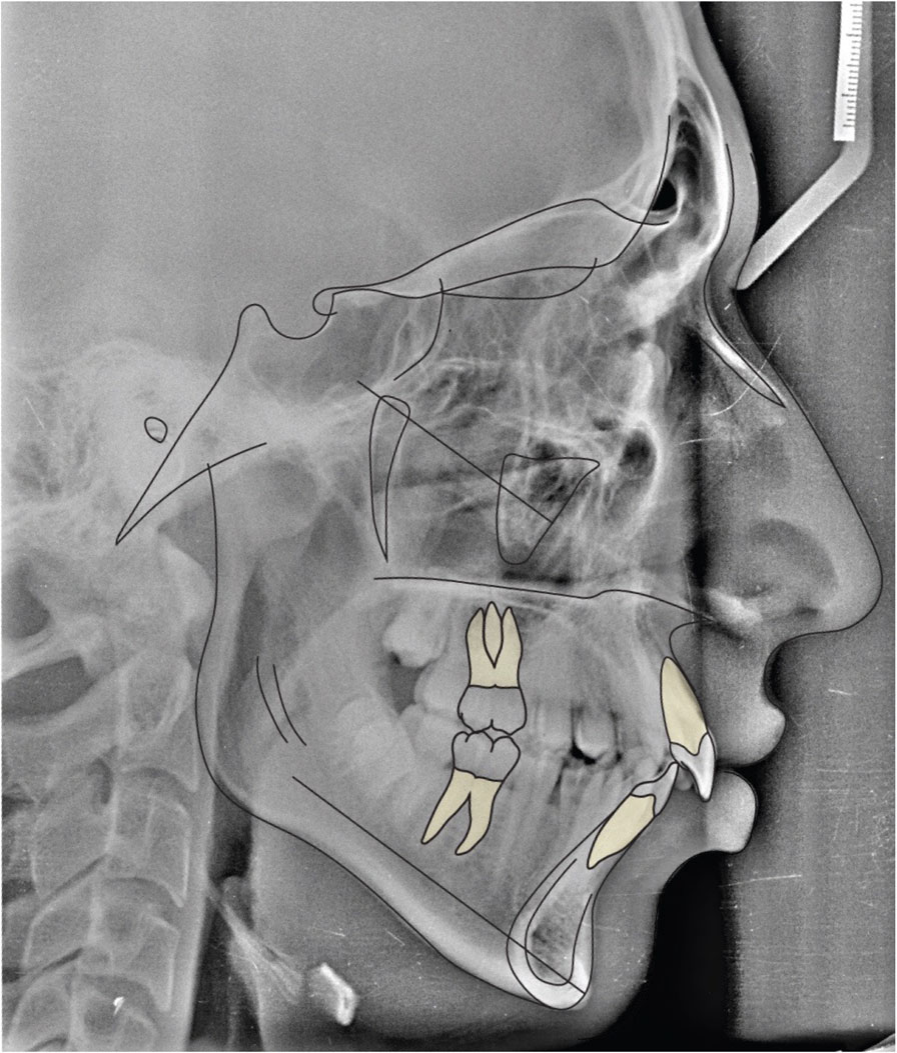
Initial radiographs and cephalometric tracing
